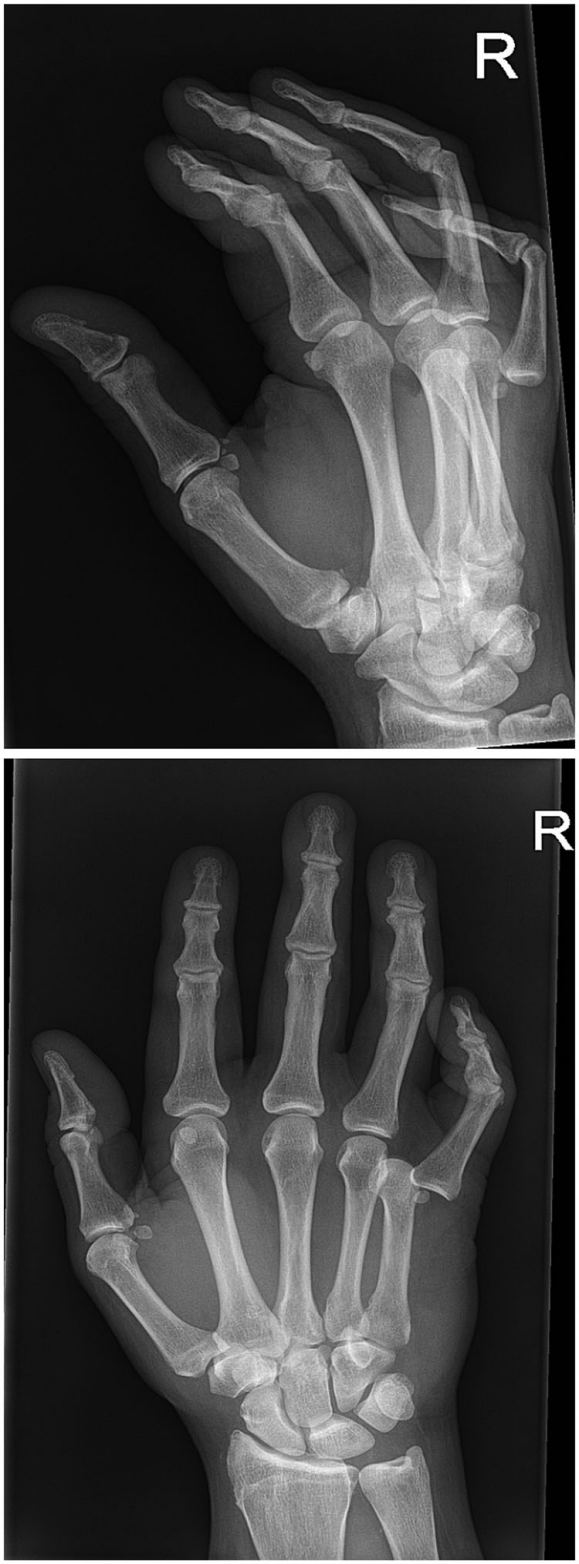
X-ray of the right hand during the examination at the ED.
